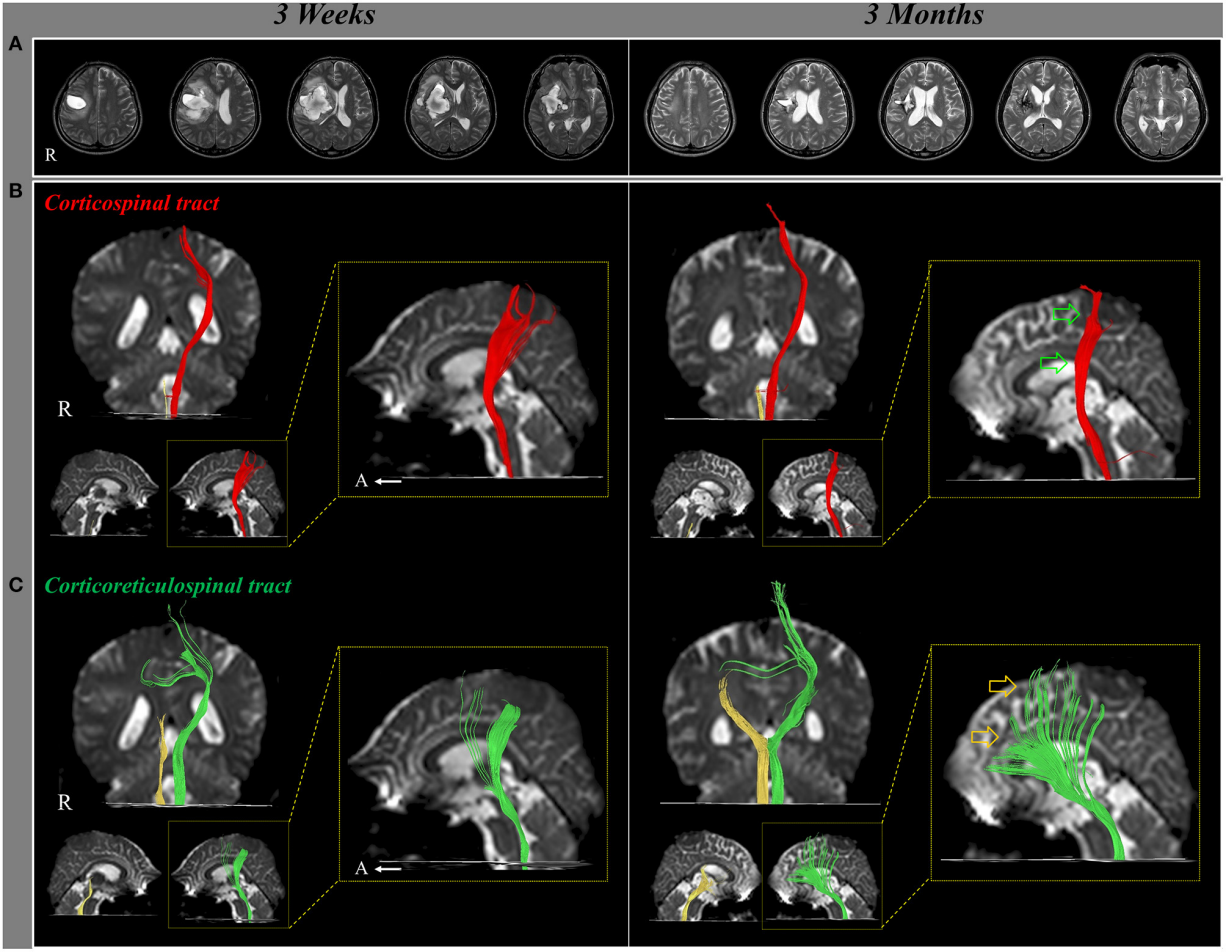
Changes of the neural tracts during the motor recovery of the paretic (left) leg. (A) T2-weighted MR images show a putaminal hemorrhage in the right hemisphere at 3 weeks and 3 months after onset. (B) Result of diffusion tensor tractography (DTT), the right corticospinal tract (CST) shows discontinuation at the brainstem on both 3-week and 3-month DTT. By contrast, on 3-month DTT, the left CST has become thinner (green arrow) compared with 3-week DTT. (C) The right corticoreticulospinal tract (CRT) shows discontinuation below the lesion on both 3-week and 3-month DTT. On 3-month DTT, the left CRT has become thicker (yellow arrow) compared with 3-week DTT (reprinted with permission from Jang and Lee, 2017).
